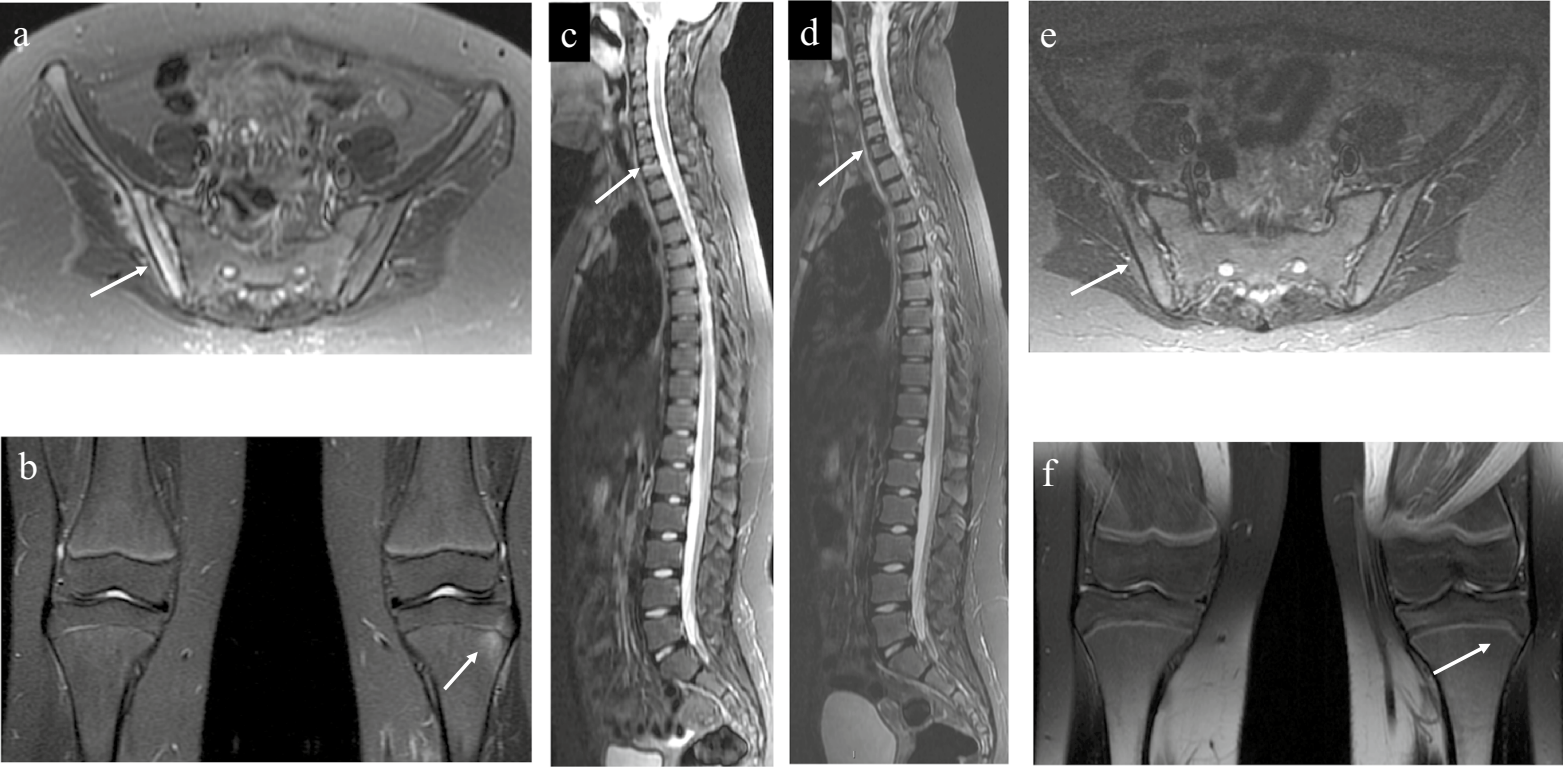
A 10-year-old girl complaining of thoracic and lumbar pain for two years who was initially treated for Langerhans cell histocytosis, despite two negative biopsies, without resolution of the pain. A WB-MRI was performed, showing bone marrow edema in the iliac portion of the right sacroiliac joint with joint effusion (a), lesion in the left proximal tibial metaphysis extending to the epiphysis (b) and edema and height loss of the vertebral body of T1 (c). The patient started treatment for CNO with non-steroidal anti-inflammatory drugs (NSAIDs) and pamidronate and showed clinical improvement. WB-MRI performed after 3 months showed stability of the T1 fracture (d) and complete resolution of the lesions of the pelvis (e) and the knee (f), without the appearance of new lesions
